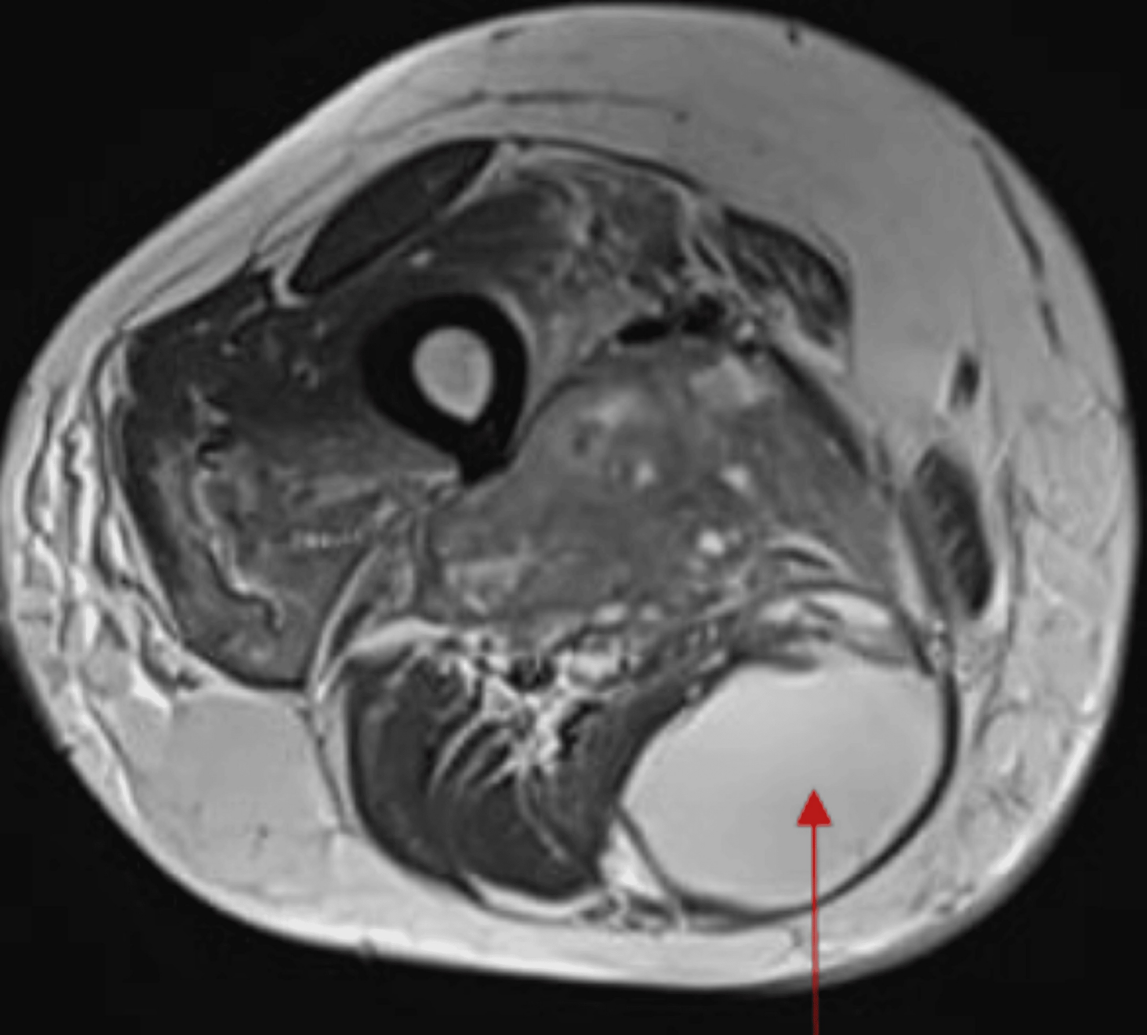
MRI of the right thigh T2-weighted image showing hyperintense collection (red arrow)
